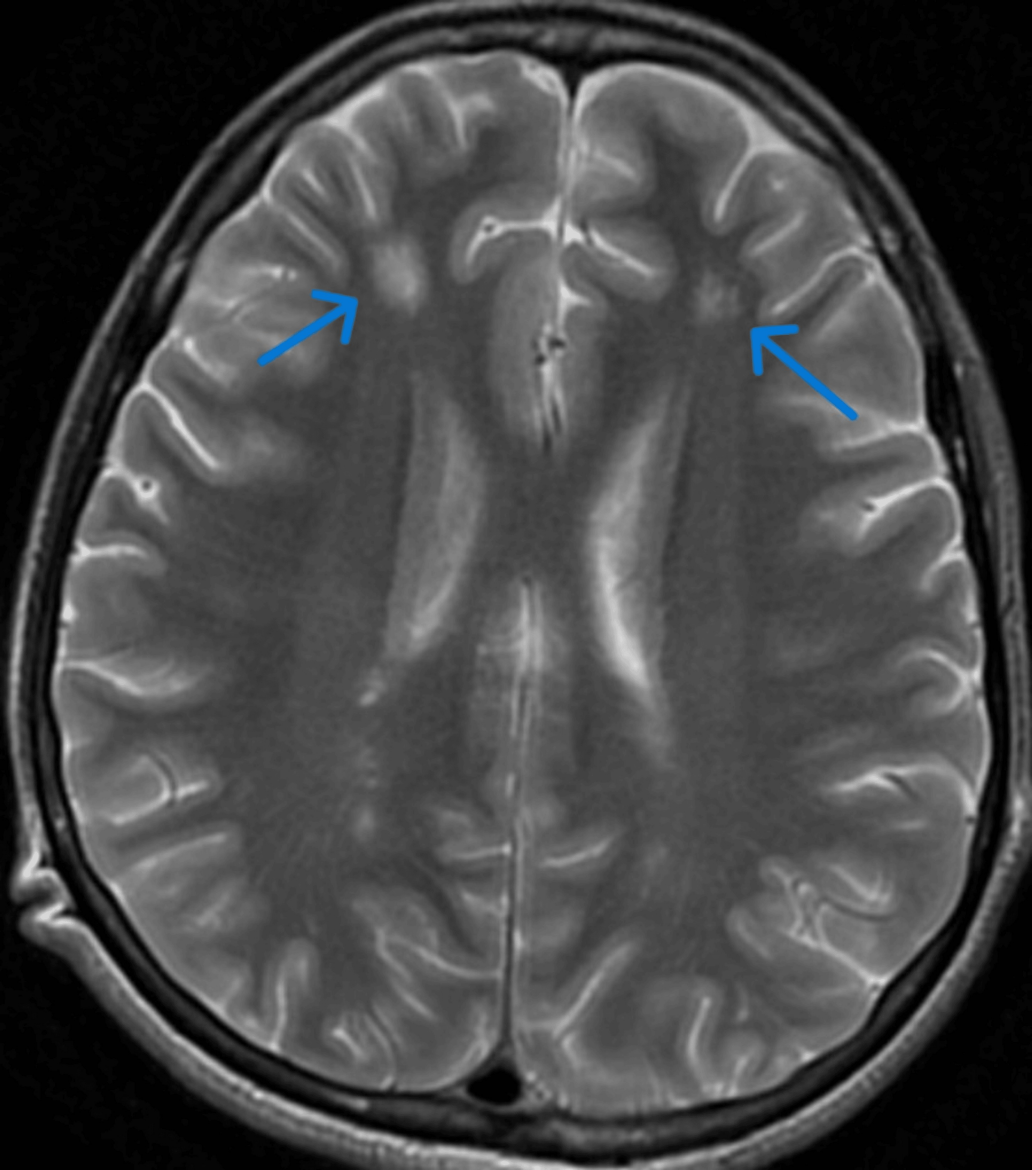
Contrast-enhanced T2-weighted MRI brain film showing frontal hyperintensities
